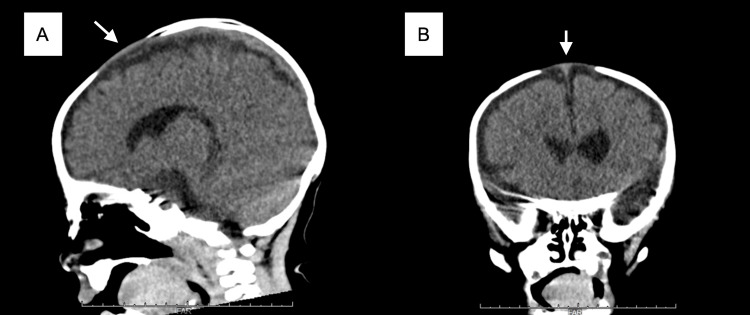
Computed tomography findings Sagittal (A) and coronal (B) reconstruction showed a bulging fontanel. The images show the projection of the large fontanel from the skull (arrows).
